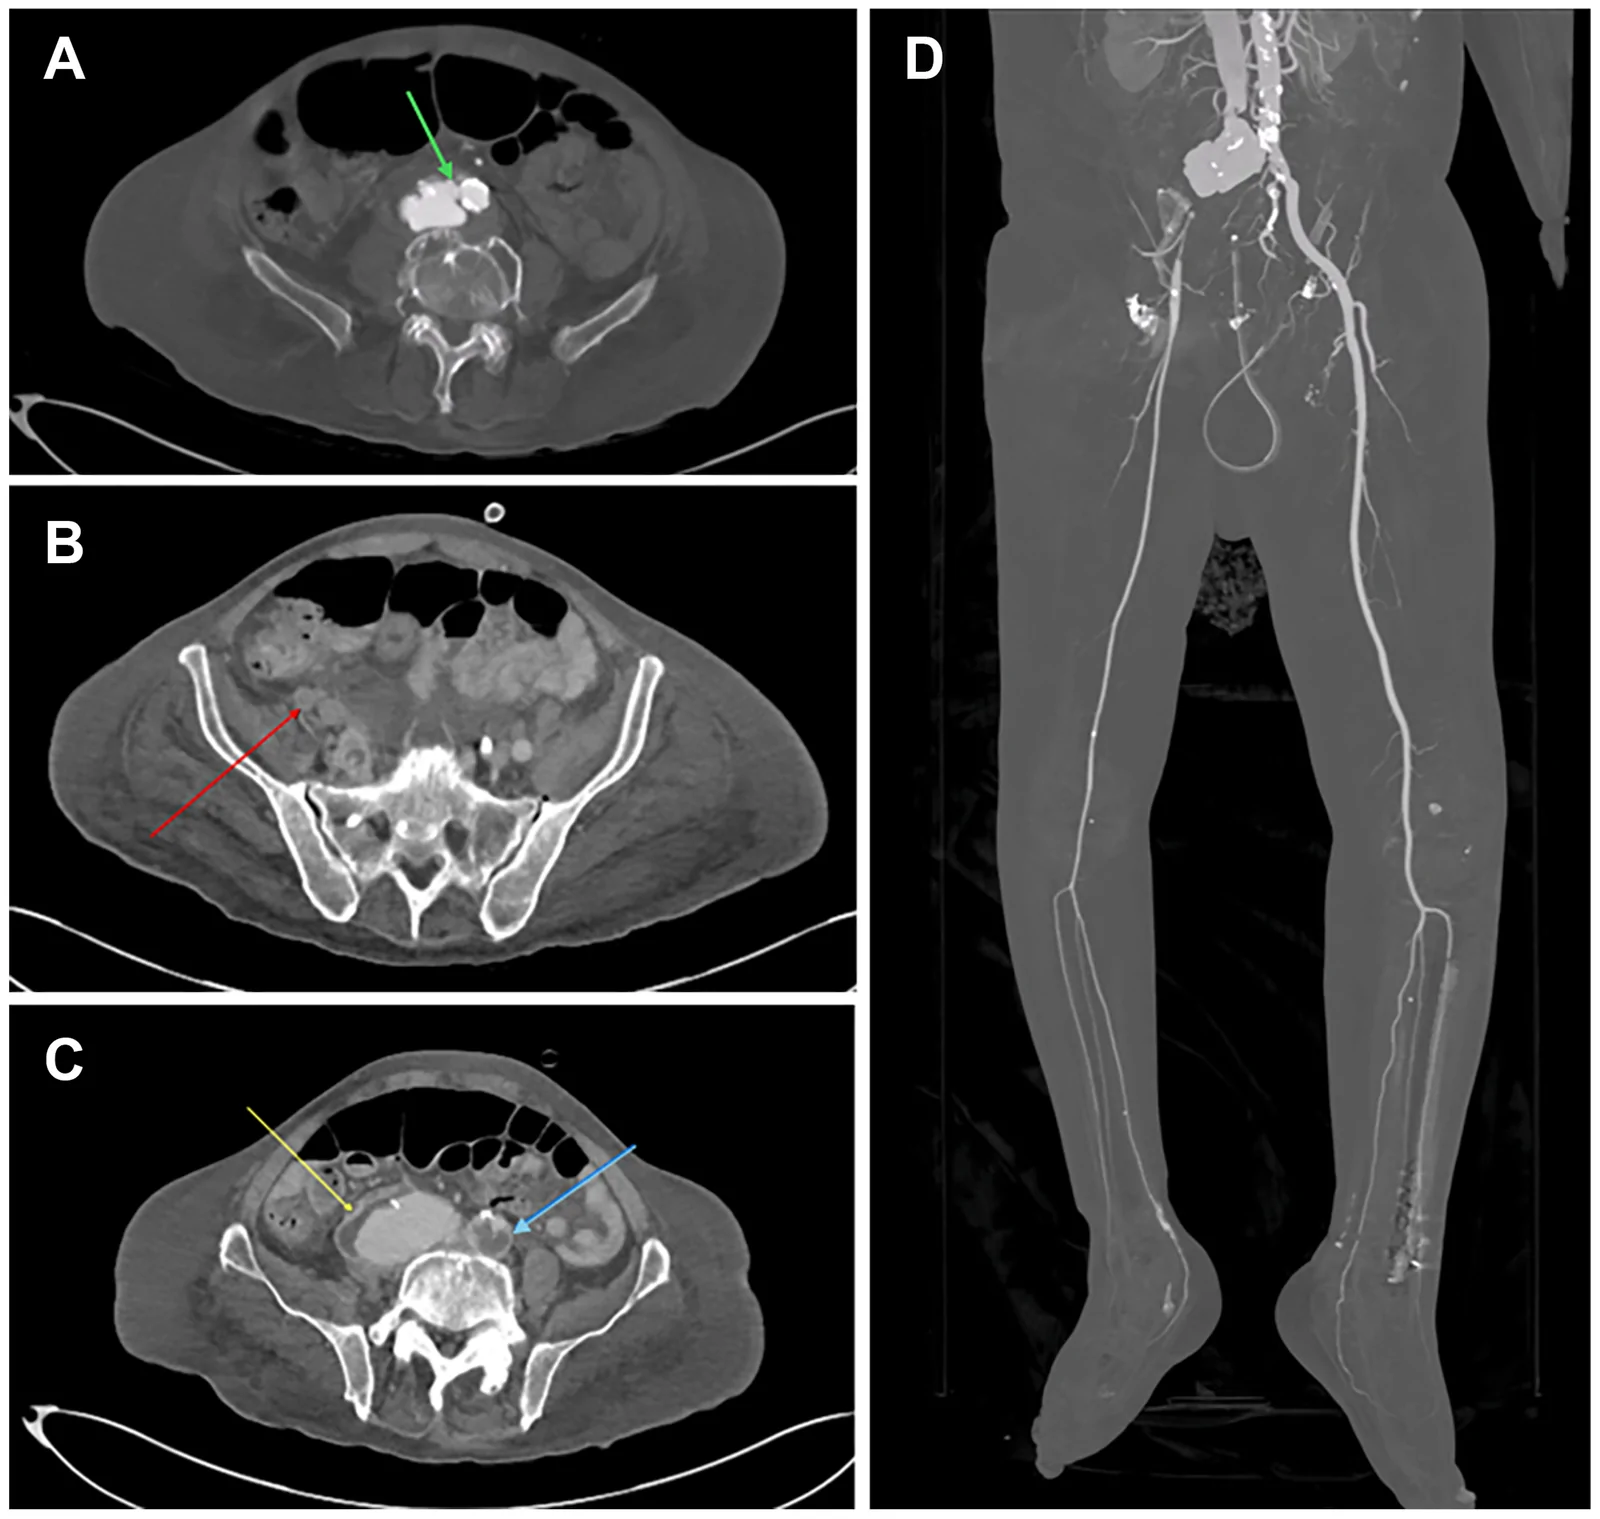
Preoperative computed tomography angiography. A, Axial computed tomography image demonstrating a fistulous communication between the aneurysmal common iliac artery and the inferior vena cava, consistent with an iliocaval fistula (*yellow arrow*). B, Computed tomography image demonstrating a right common iliac artery aneurysm with mural thrombus and contained rupture. The right external iliac artery is occluded by thrombus (*red arrow*). C, Computed tomography image demonstrating degenerative aneurysmal change of the distal left common iliac artery near the iliac bifurcation (*blue arrow*) and a right common iliac artery aneurysm with mural thrombus (*yellow arrow*). Diffuse aneurysmal and atherosclerotic changes are also present. D, Maximum intensity projection image demonstrating the overall arterial anatomy and diffuse luminal narrowing of the arterial tree in the right lower extremity.
